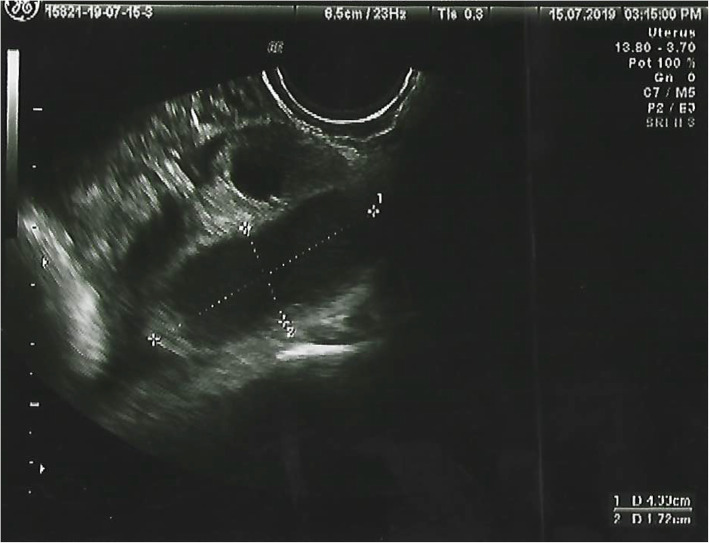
Pelvic ultrasound showing hydrosalpinx and normal left ovary
